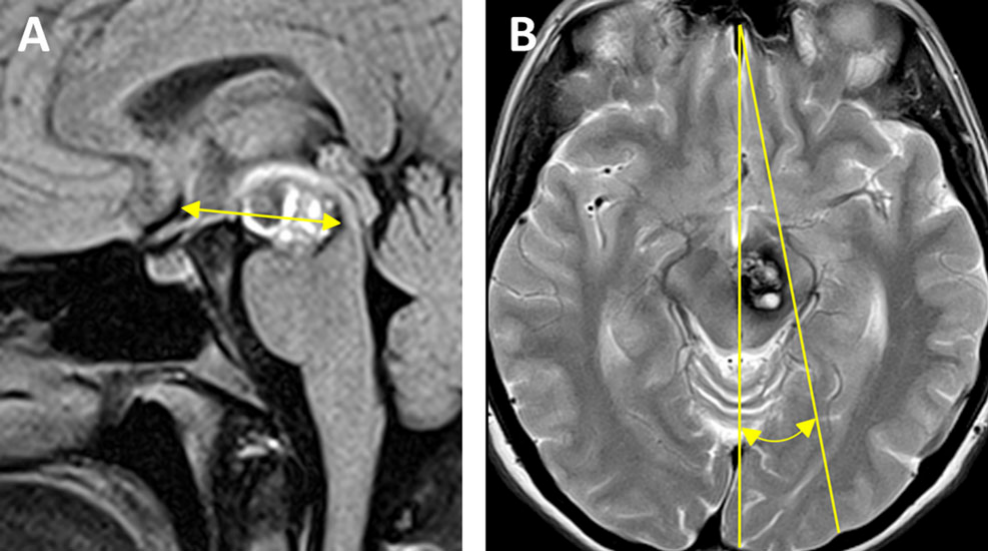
Definition of the tumor *depth* and *laterality angle*. a Tumor depth was defined as the distance between the anterior communicating artery and posterior tumor margin on the sagittal MRI slice with the largest tumor extent. b Tumor laterality angle was determined as the geometric angle between the sagittal midline and a line connecting the anterior margin of the frontal lobe in the midline with the most lateral tumor margin on the axial MRI slice with the largest tumor extent
